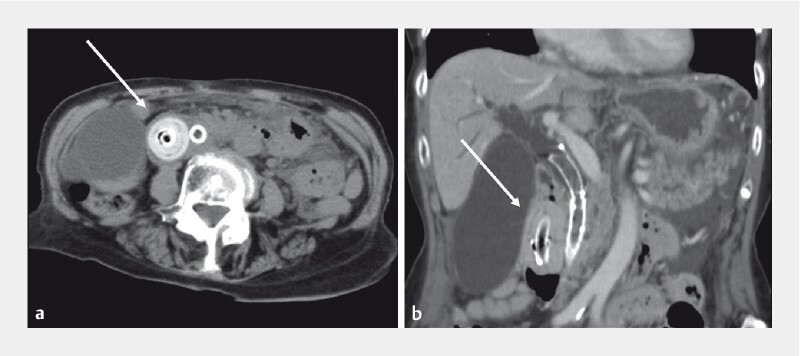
Contrast-enhanced computed tomography scans showing the calcified stent-stone complex (white arrows) formed at the end of the stent on the stomach side and pulled into the duodenum.
